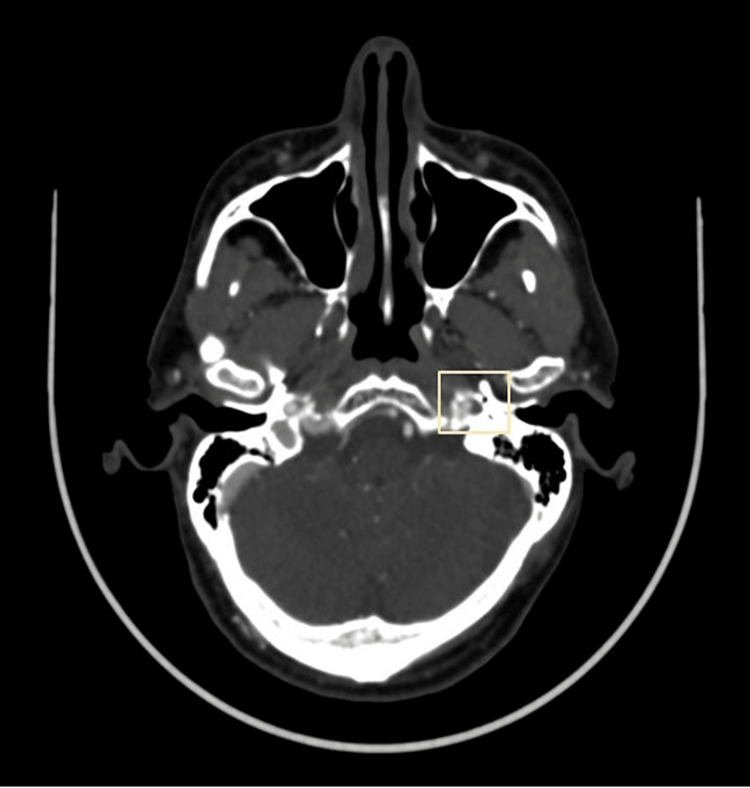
CT angiography showing a narrow opacified true lumen with a crescent sign suggestive of false lumen due to dissection.
